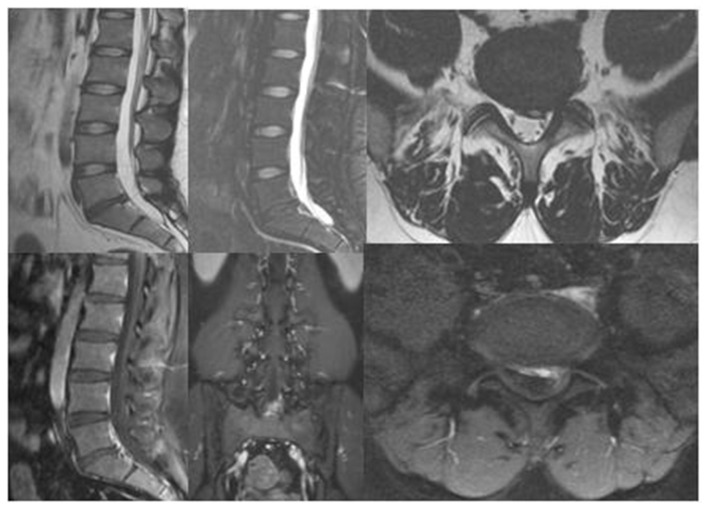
Follow-up lumbar MRI of the patient 6 years later, demonstrating significant reduction, nearly complete disappearance, of the L5/S1 disc herniation. The patient's symptoms had resolved. The rim enhancement observed on the contrast-enhanced MRI had also disappeared. The resorption rate at this point reached 83.6%.
